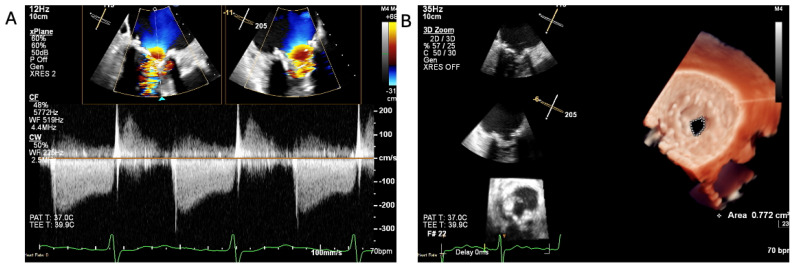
Transoesophageal echocardiography image of degenerated mitral valve bioprosthesis. (A): CW Doppler across stenotic mitral valve with mean gradient of 12 mmHg, (B): 3D planimetry mitral valve area (MVA); value of 0.77 cm^2^ in keeping with severe MS.
